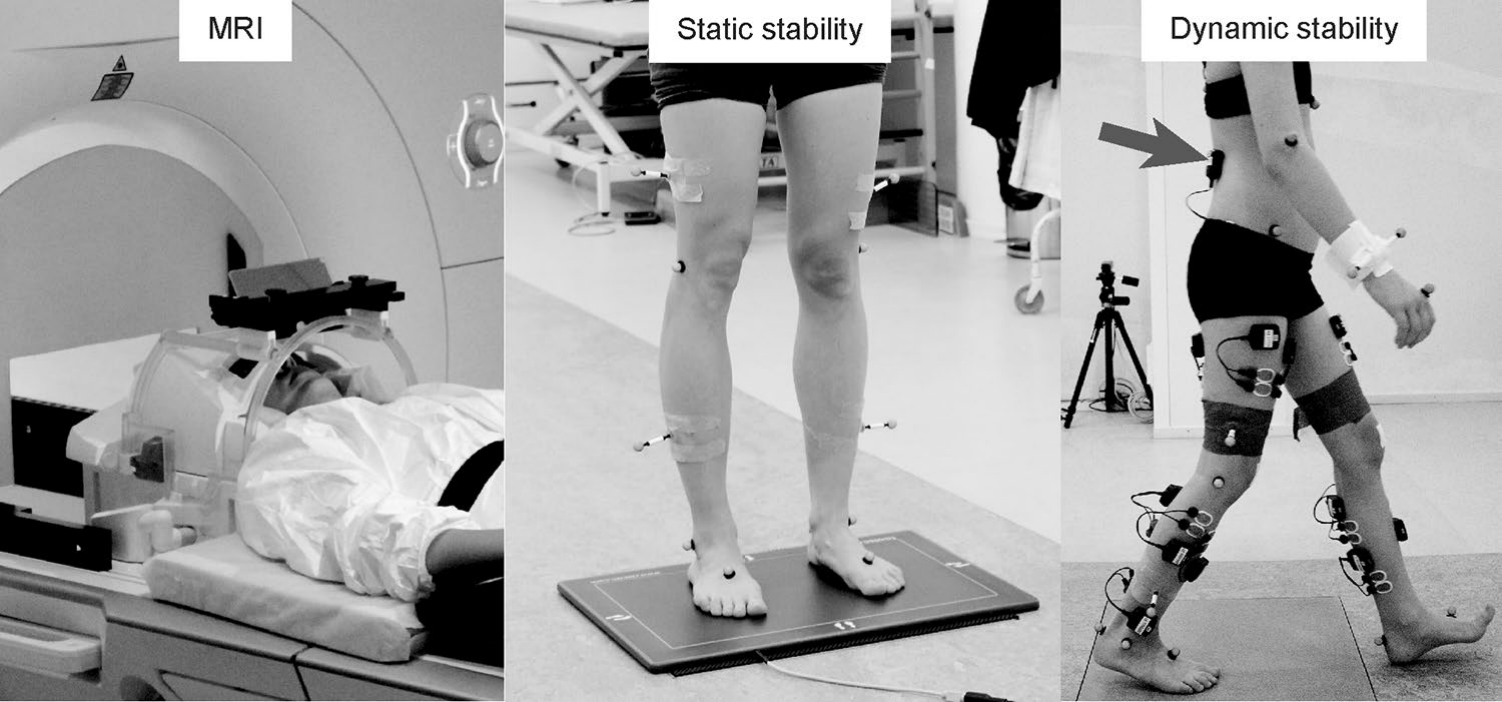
Experimental setups in MRI, static stability and dynamic stability measurements. The arrow indicates the location of inertial measurement unit on the lower back of the subject during dynamic gait stability testing
